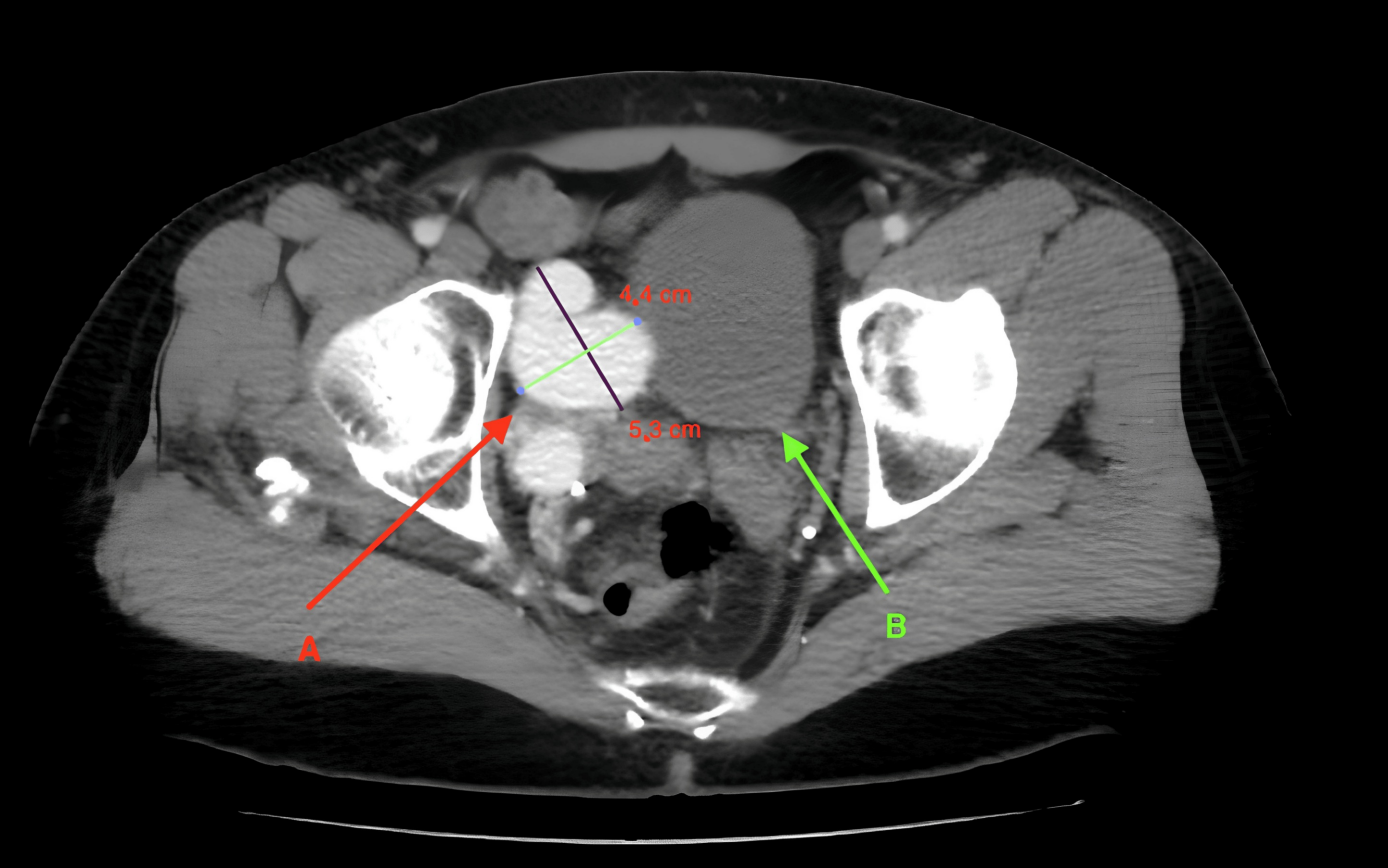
CT angiography. A horizontal section at the level of the lesser pelvis. Note the irregular aneurysmatic confluent branch of the right internal iliac vein (A) with a maximum diameter of 5.3 cm and the displacement of the right surface of the bladder (B) toward the midline.
